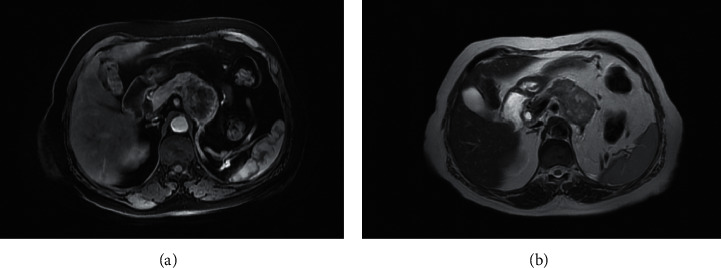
MRI images of T1 fat saturation sequences with gadolinium injection in arterial (a) and portal (b) phases showing a tissular corporeal pancreatic mass, with low signal, enhanced in periphery, comprising a necrotic center with no involvement of the superior mesenteric artery.
